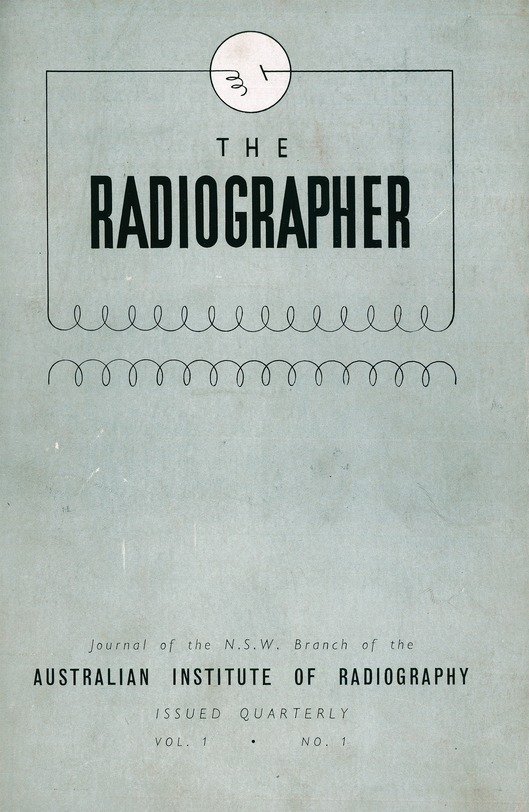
Australia: 1948 cover page of *The Radiographer*.
